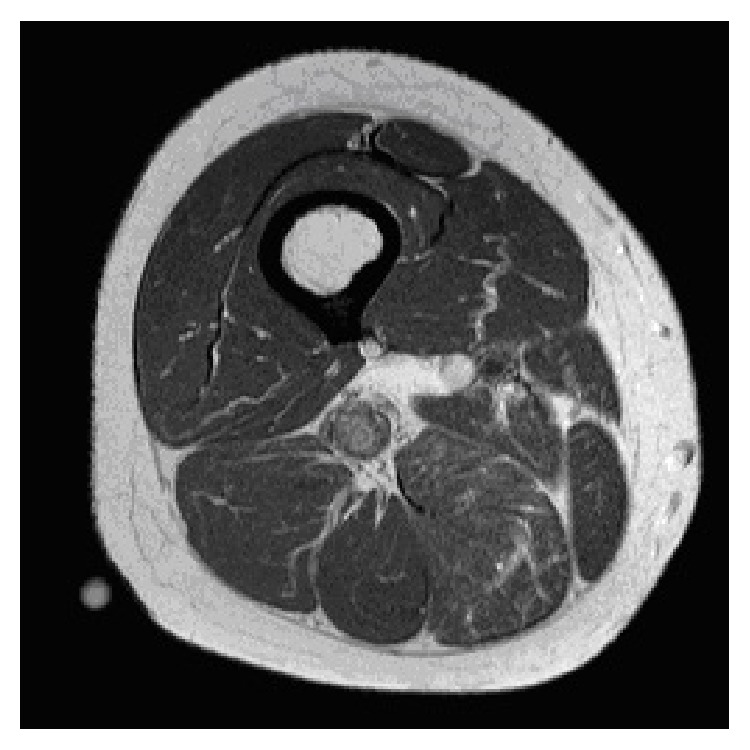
Axial MRI imaging of the right thigh demonstrating the mass originating from the sciatic nerve.
